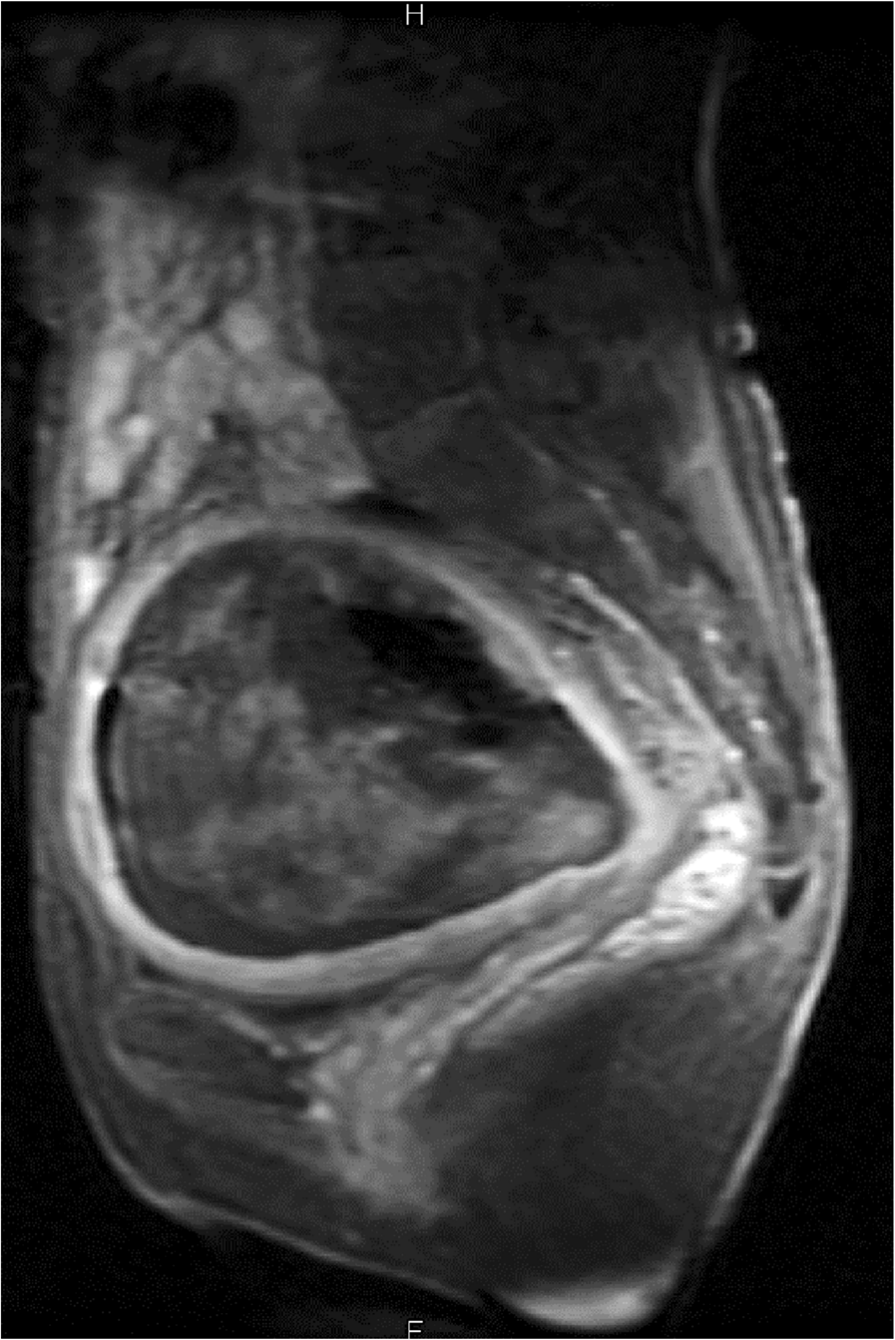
The finding of pelvic MRI at surgery of the uterine rhabdomyosarcoma. The sagittal section of an MRI showed a heterogeneously enhanced huge mass, measuring approximately 15 × 10 cm in diameter, arising possibly from the uterine corpus and, completely filling the lumen of markedly enlarged uterus
